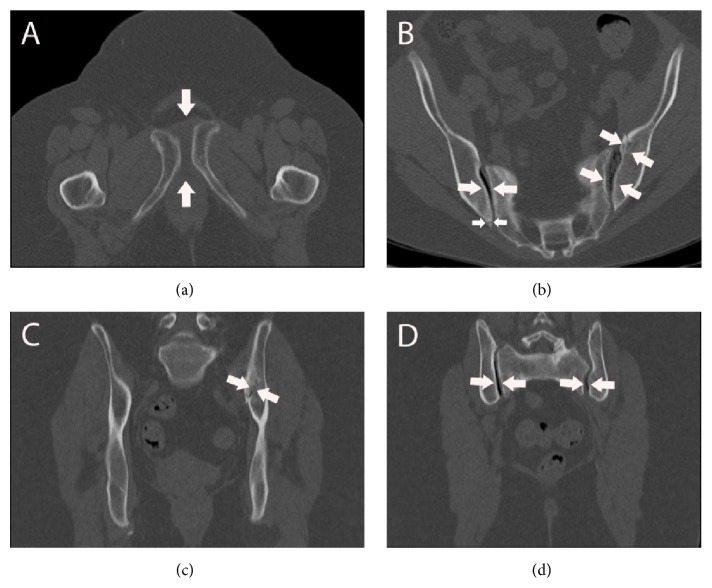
The CT-scan of the pelvic girdle confirms the observations of MR-image. (a) Axial CT-scan presented widening of the symphysis (arrows). (b) Axial CT-scan offered vacuum phenomenon in both sacroiliac joints, small tearing out in the left sacroiliac joint, and small dorsal fracture fragment in the right dorsal ilium (arrows). (c) Coronary CT-scan revealed the fracture line in the left ilium (arrows). (d) Coronary CT-scan presented vacuum phenomena in both sacroiliac joints (arrows).
